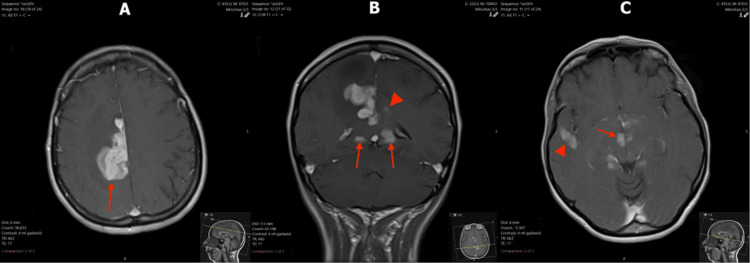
T1 post-contrast MRI images of the brain (A) Axial image with an avidly enhancing right cingulate gyrus/frontoparietal lesion with surrounding vasogenic edema (arrow). (B) Coronal image demonstrates a well-defined, homogeneously enhancing lesion involving the right cingulate gyrus with surrounding vasogenic edema. Additional bilateral lesions adjacent to the occipital horns of both lateral ventricles (arrows) and trace enhancement of the left cingulate gyrus (arrowhead) are also depicted. (C) Axial image with enhancing lesions in the right temporal lobe (arrowhead) and abutting the third ventricle (arrow). MRI: magnetic resonance imaging
